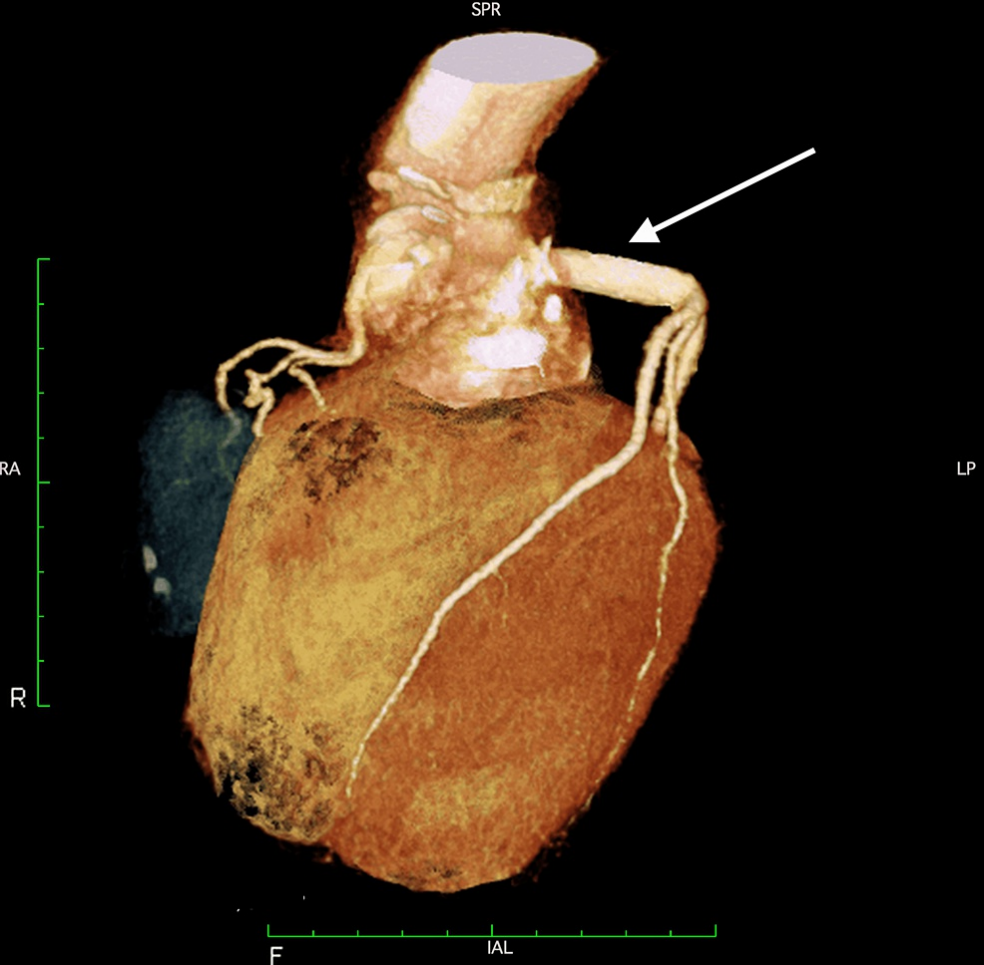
A postoperative contrast-enhanced computed tomography The coronary artery was reconstructed with the artificial graft (arrow) without any complications of the anastomosis site.
